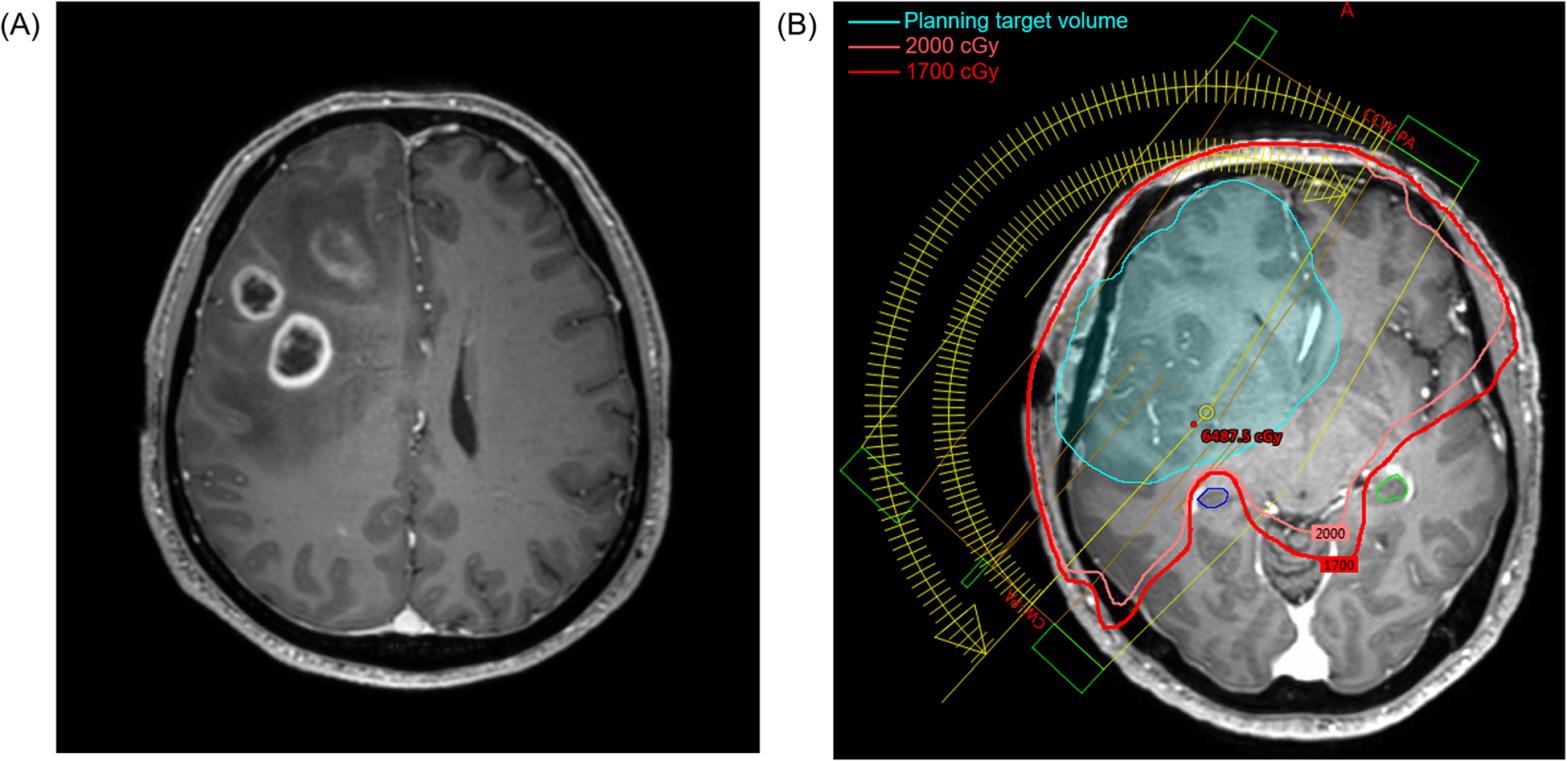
A 65-year old man showing multiple enhancing lesions involving the right frontal lobe, corpus callosum body/splenium, and right parietal lobe on (a) preoperative magnetic resonance imaging. The patient was pathologically confirmed as *IDH1* wild-type glioblastoma with methylated *MGMT* promoter after receiving partial resection of the tumor followed by temozolomide-based chemoradiotherapy of 60 Gy in 30 fractions. b Using 2 partial arcs, the maximum dose to the right (ipsilateral, blue contour) and left (contralateral, green line) hippocampi were 16.93 Gy and 16.38 Gy, respectively, on a volumetric-modulated radiotherapy plan. Abbreviations: *IDH1*, Isocitrate dehydrogenase 1; *MGMT*, O^6^-methylguanine-DNA methyltransferase
